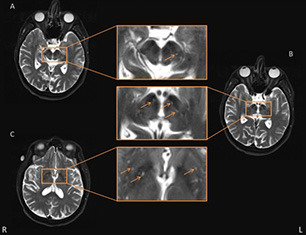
Representative axial T2-weighted images showing basal ganglia (BG) and midbrain perivascular space (PVS). Some typical PVS are indicated by the orange arrow. Panels (A,B) are two consecutive layers showing PVS in the midbrain, in which the PVS in panel (A) is continuous to the rightmost PVS in panel (B), and the PVS is shown passing through the SN. The other PVS in panel (B) were also deemed adjacent to SN. Panel (C) shows the axial slice which had the most PVS in the BG area.
